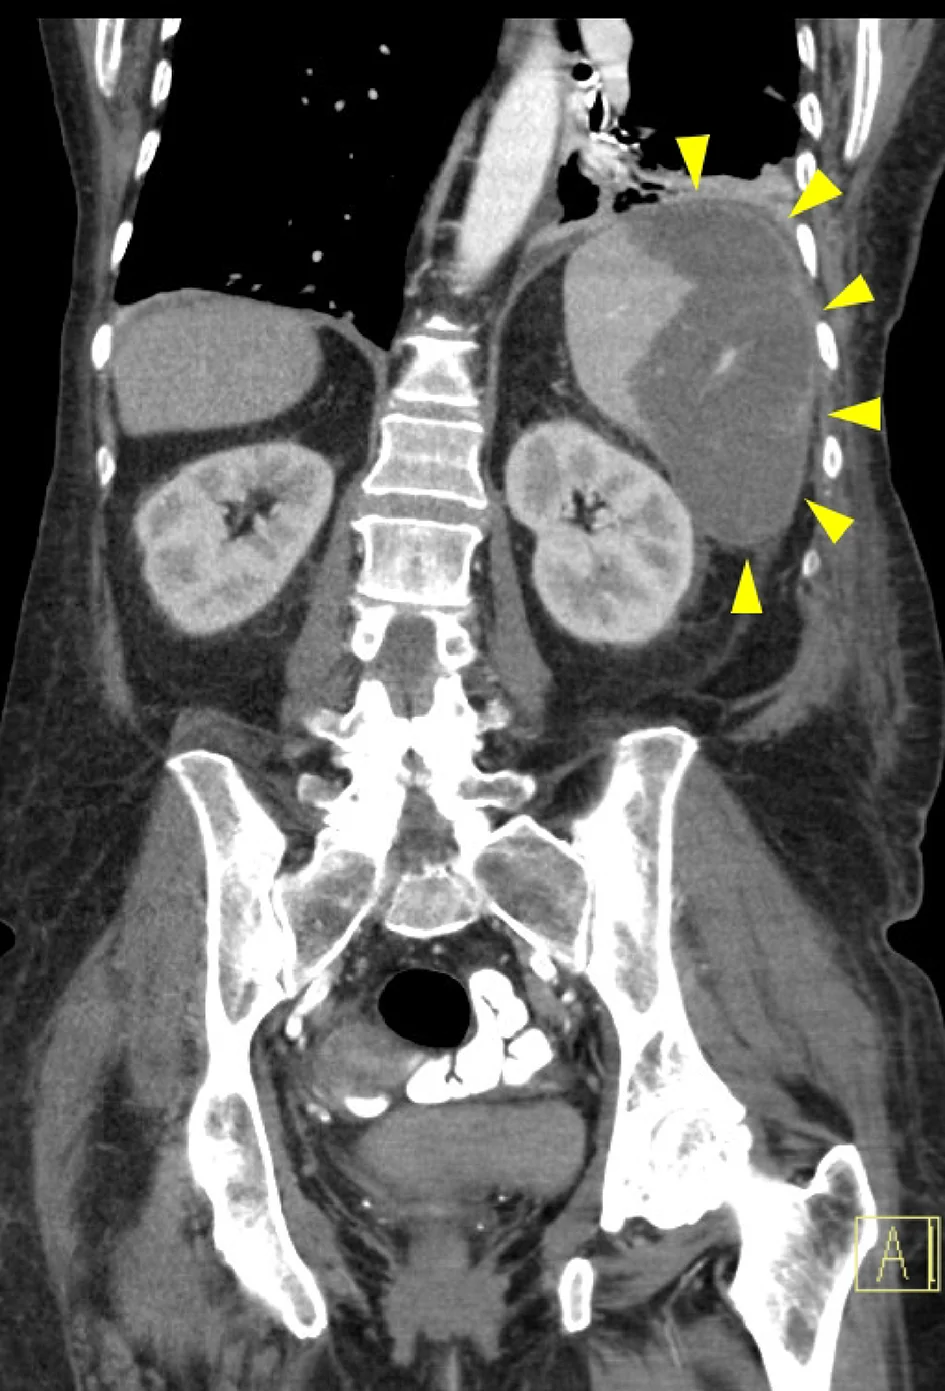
Computed tomography angiography showed extensive splenic infarction with near-complete involvement of the splenic parenchyma (arrowheads)
